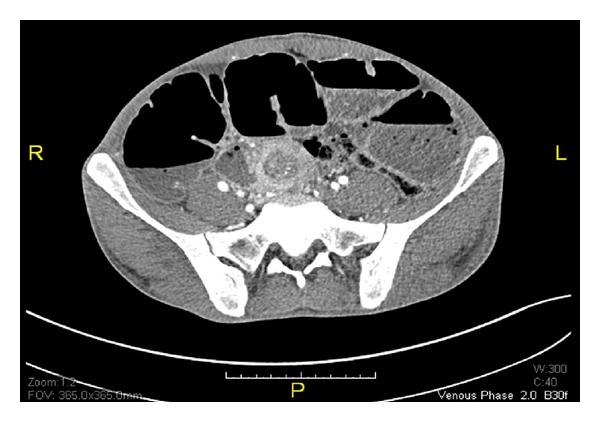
Abdominal CT scanning on day 19 (day 15 after symptoms) showing severely dilated small bowel and characteristic intussusception features: “target lesion” or “doughnut sign” and sausage-shaped mass.
